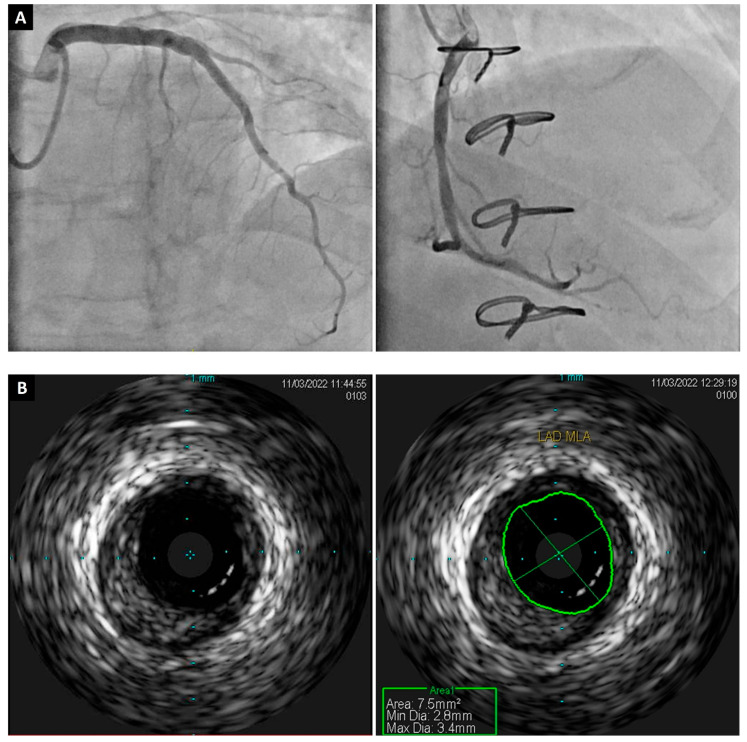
A 62-year-old male patient who developed CAV 10 years after heart transplant. (A) Conventional ICA showing no significant stenosis; middle left anterior descending artery is 10% obstructed by diffuse disease and middle right coronary artery is 20% obstructed by diffuse disease. (B) IVUS was performed in mid left anterior descending artery and confirmed the presence of significant atherosclerosis. *CAV, cardiac allograft vasculopathy; ICA, invasive coronary angiography; IVUS, intravascular ultrasound*.
